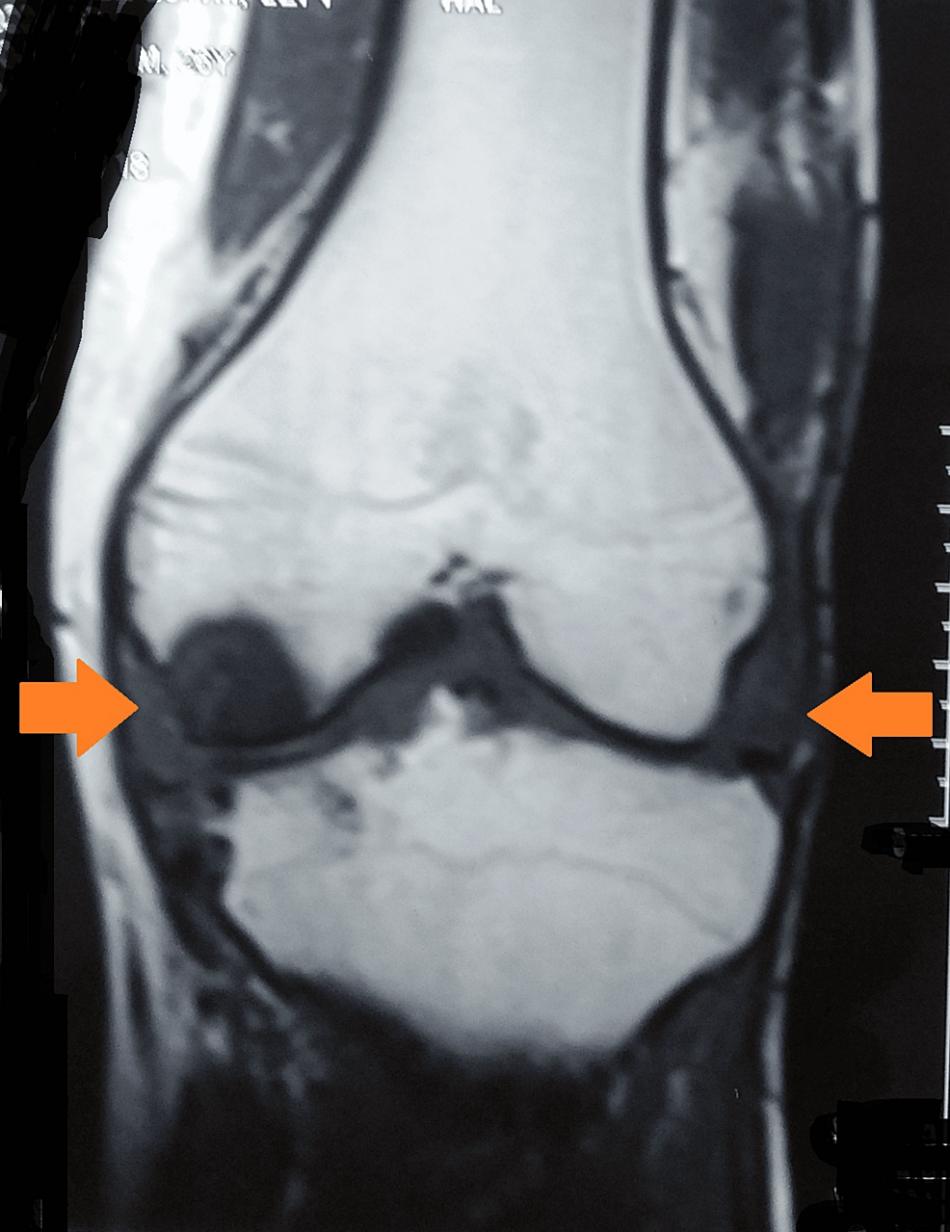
MRI of the left knee joint suggestive of knee joint involvement MRI: magnetic resonance imaging
